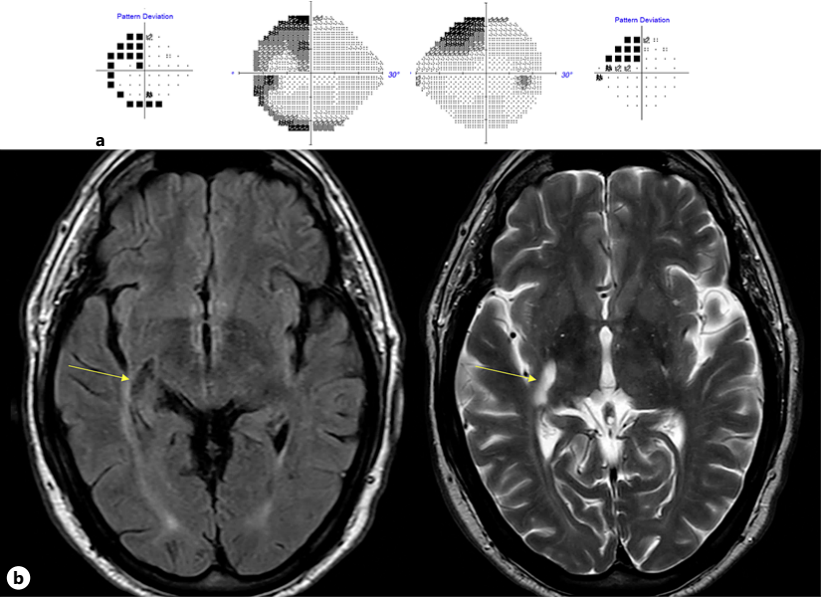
a Humphrey 24-2 SITA-Fast visual field testing showing a left superior homonymous quadrantanopia. b MRI FLAIR (left) and T2 demonstrating a 2.0 × 0.5-cm oblong-shaped cerebrospinal fluid-filled space posterior to the right basal ganglia, just above the temporal horn of the lateral ventricle, which courses close to the expected location of the lateral genicular body. A thin rim of FLAIR hyperintensity is noted at the margin of the lesion. This was thought to represent a giant Virchow-Robin space.
